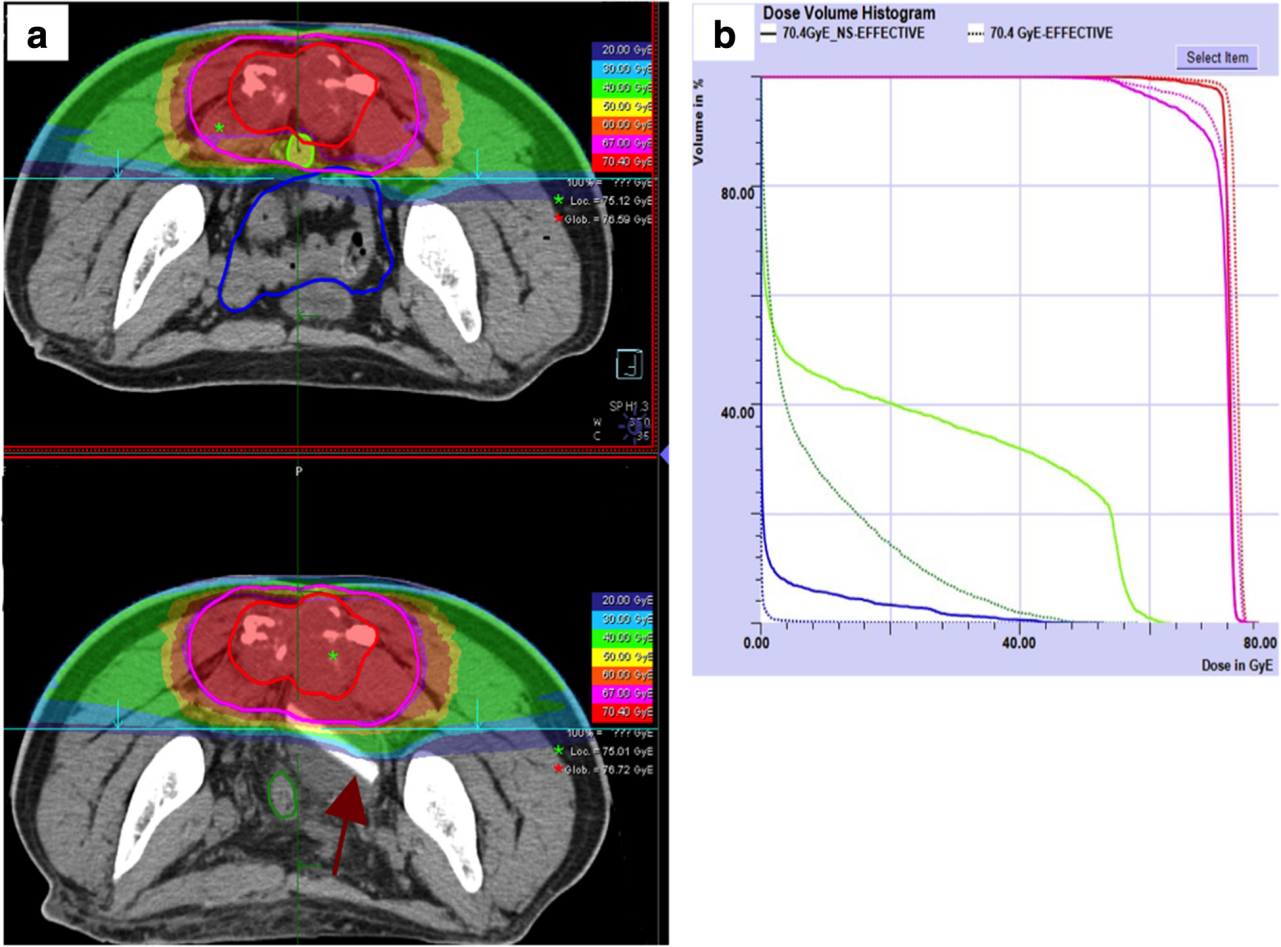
Plan comparison study on different CT from the same patient selected for spacer positioning at CNAO. a Different CIRT plans depicted on axial images at the same anatomical level for the same chordoma patient. *Red lines* for GTV and *green lines* for CTV are included within the prescription dose isodose in both CIRT plans of 70.4 Gy (RBE). In the *upper panel*, the digestive area (rectal wall in *green* and intestine in *blue*) is contiguous to the prescribed dose isodose. In the *lower panel*, the digestive tract is far away from irradiated area due to the spacer (pointed by a *red arrow*). b Comparison of DVH for GTV (*red*), PTV (*pink*), and digestive tract (rectum *green*, intestine *blue*) dose coverage from the two CIRT plans compared in a. Coverage of the tumor is higher with the spacer CIRT plan (*dotted lines*) compared to the plan without it (*continuous lines*). With the CIRT spacer, plan dose sparing to the digestive tract is achieved
